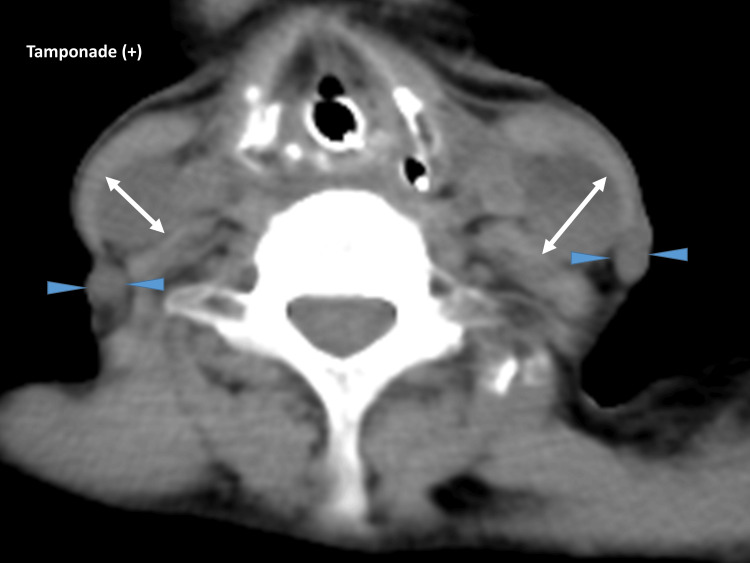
Neck computed tomography. Example of the measurement of the diameter of the right and left internal jugular vein at the vocal cord portion in a patient with cardiac tamponade (arrowhead, external jugular vein; arrow, internal jugular vein).
